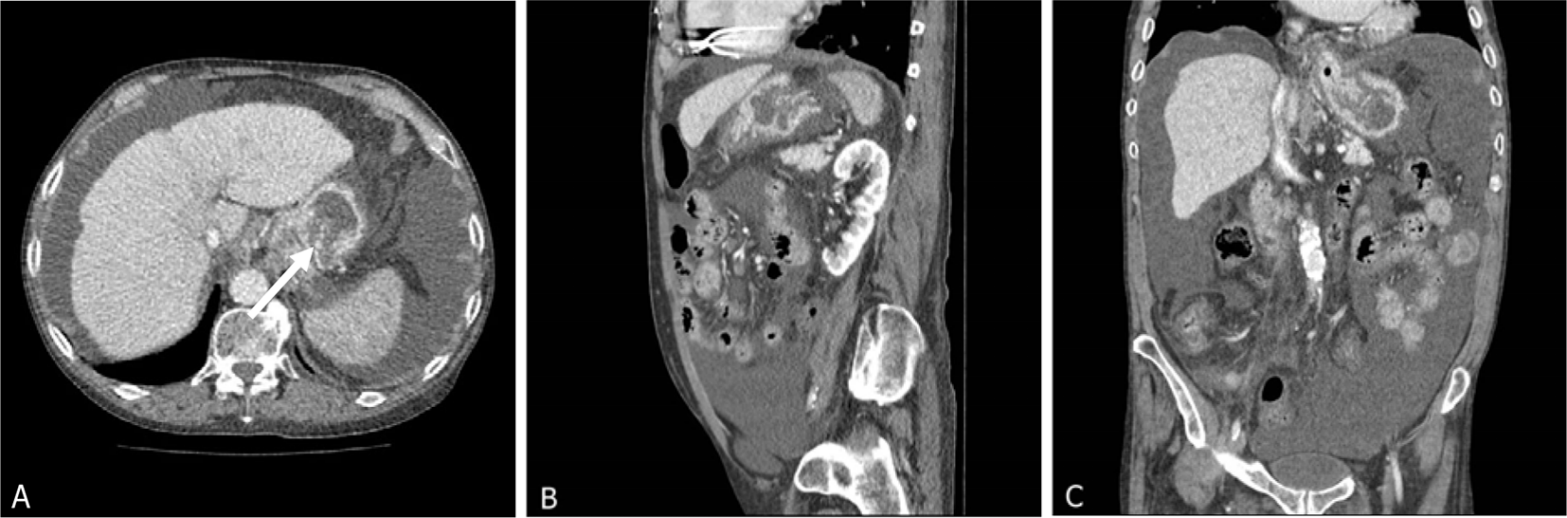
CT abdomen and pelvis March 2017 with evidence of ascites, gastrogastric intussusception and vascular pseudopedicle (white arrowhead).
